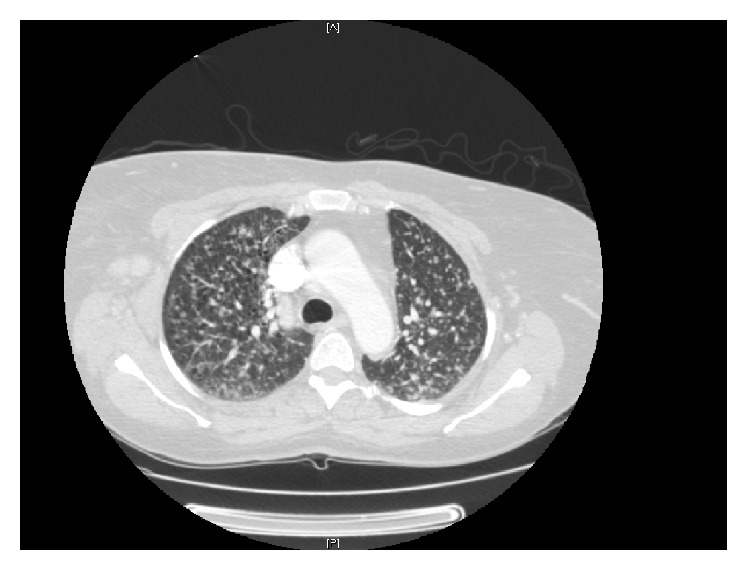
CT Scan of the chest showing miliary pulmonary nodules.
